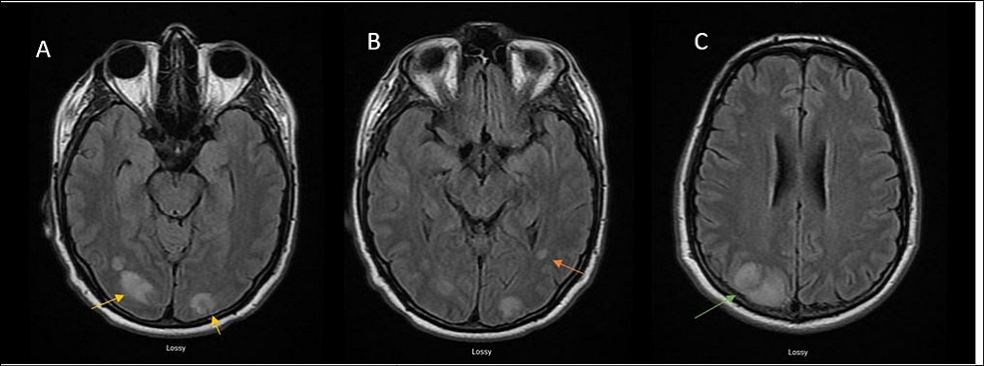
MRI of the brain showing multifocal lesions involving the bilateral occipital lobes (yellow arrow in A), parietal lobes (orange arrow in B), and cerebellum (green arrow in C) consistent with multifocal abscesses with edema. MRI: magnetic resonance imaging
